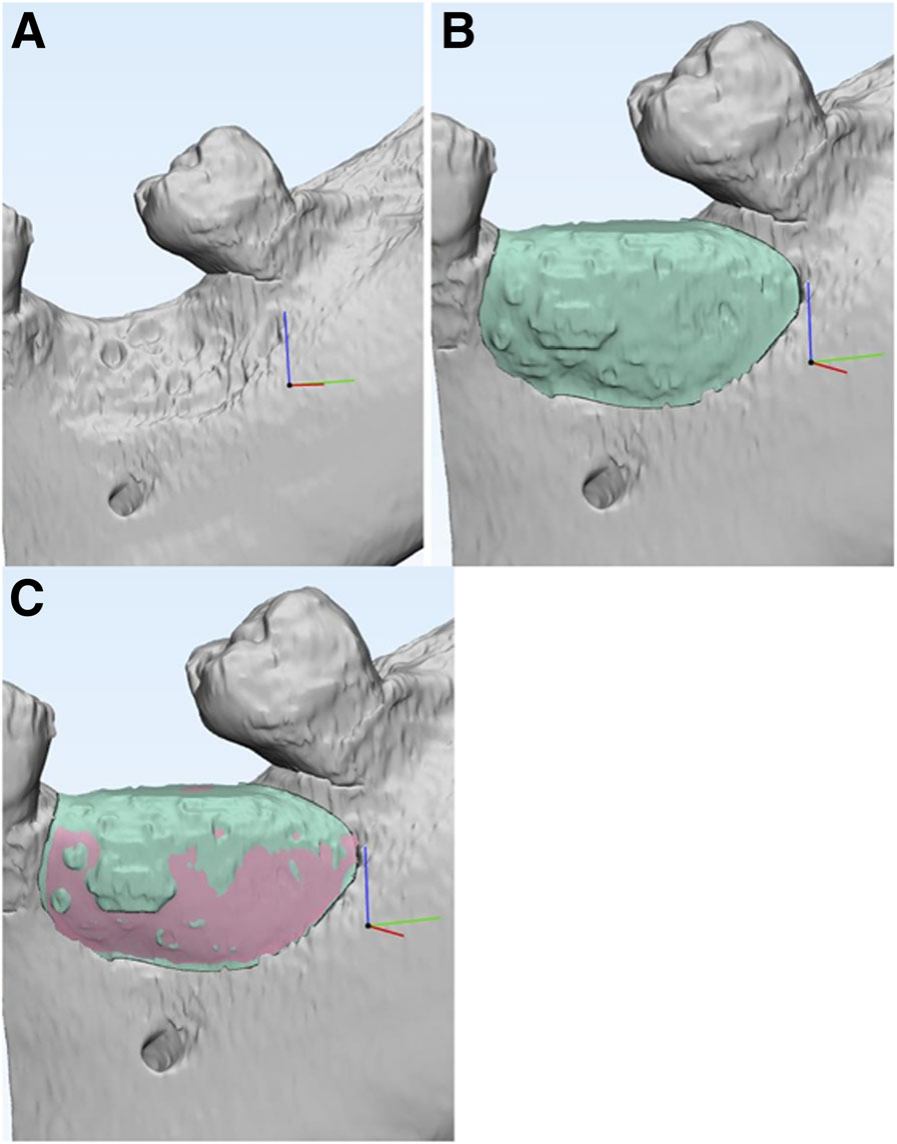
a 3D reconstruction of baseline CBCT. b Global registration of pre- and post-operative 3D reconstructions; new bone shown in green. c Percentage bone fill calculated by comparing planned augmentation volume (pink) with the volume of new bone (green)
